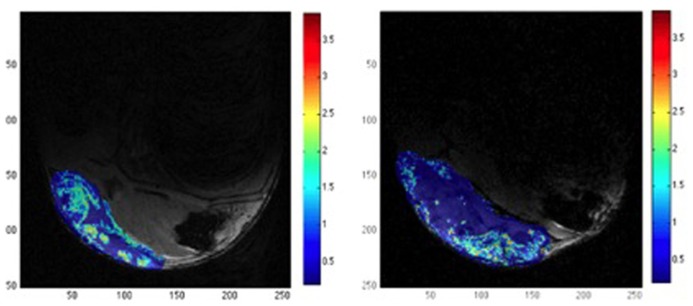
Example of relative tumor blood volume rTBV (color) overlaid on a structural MR image (gray level). The images show the effect of DMOG treatment that affects angiogenesis process (left) versus placebo (right). DMOG treated tumor shows multiple small regions with relative high rTBV, while placebo treated tumor present only one big region with significant rTBV. The color bar indicates the rTBV values in arbitrary units. Adapted from Dominietto et al. (2012), reproduced with permission.
